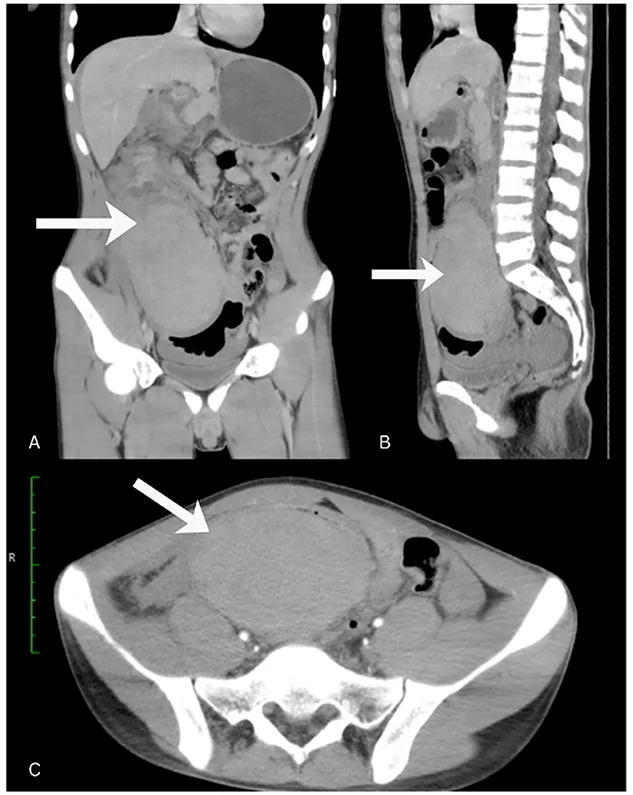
Contrast-enhanced computed tomography (CECT) of the abdomen showing an intramural hematoma (arrow) of the cecum and ascending colon measuring 7.9 cm AP × 9.8 cm TR ×14.4 cm long; (A) coronal view, (B) sagittal view, and (C) axial view.
